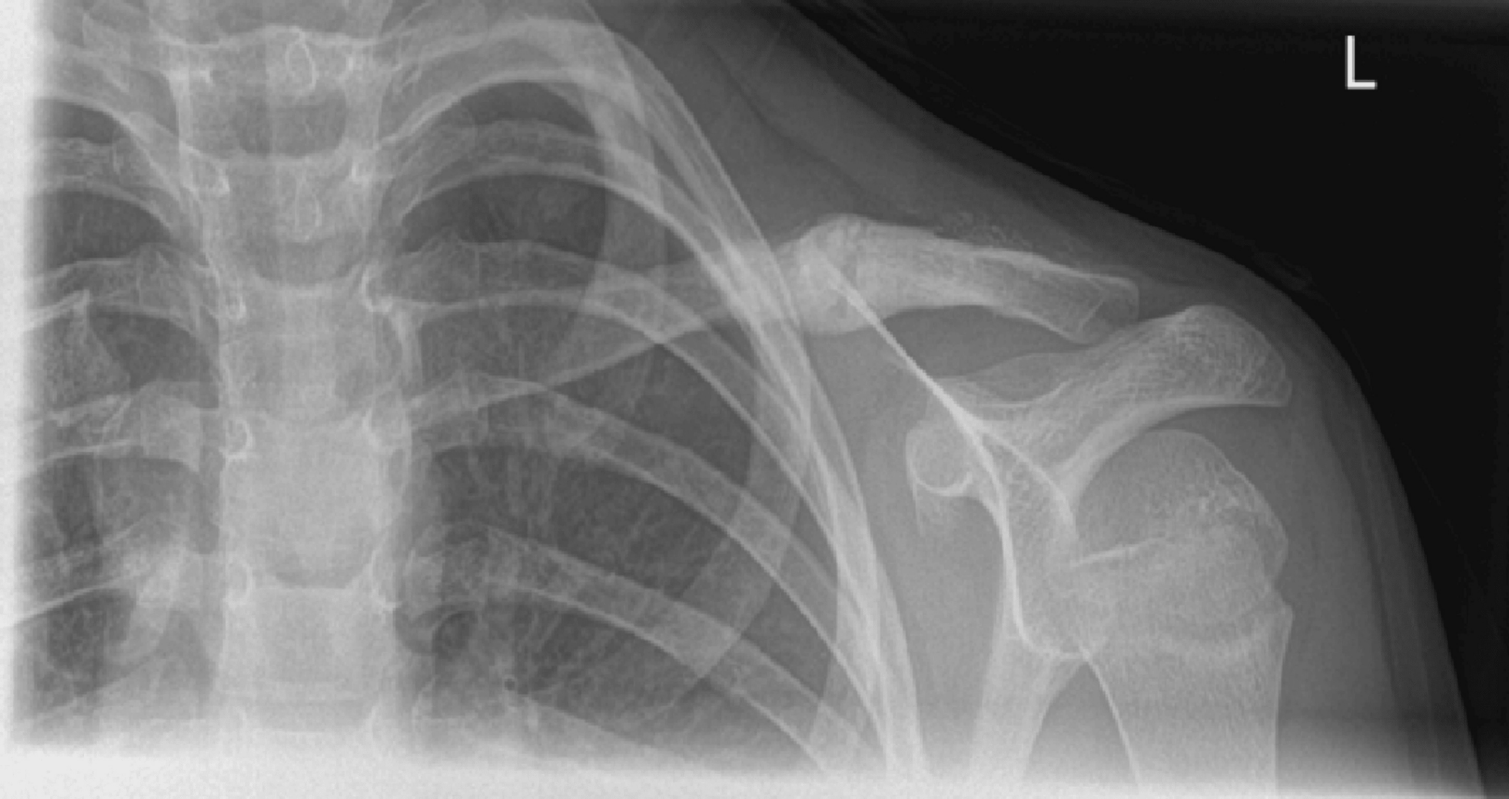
Anteroposterior radiograph demonstrating a reactive callus in the left clavicle
